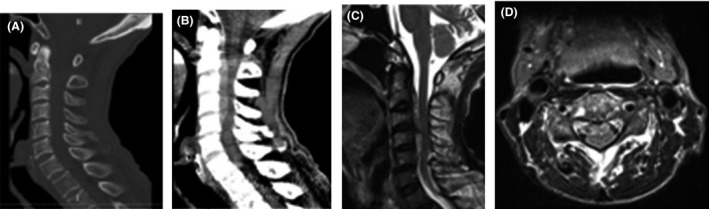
Imaging findings from case 1, an 82‐year‐old Japanese man who suddenly developed dysstasia without back or neck pain. A, B, Bone‐specific computed tomography reveals no definite findings, although a high‐density soft tissue mass is visible in the spinal canal at C2–C5. C, D, Sagittal and axial T2‐weighted magnetic resonance imaging reveals a spontaneous spinal epidural hematoma on the ventral side of the spinal cord at C2–C5.
